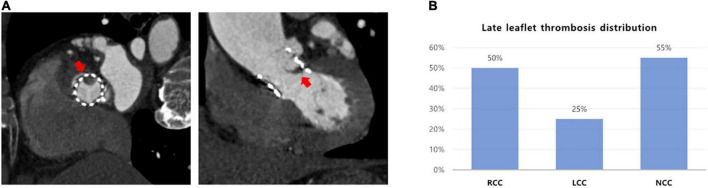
The distribution of subclinical leaflet thrombosis in TAVR. (A) Evaluation of leaflet thickening and reduced leaflet motion based on MDCT. (B) Leaflet thrombosis distribution in cusps. MDCT, multidetector computed tomography; TAVR, transaortic valve replacement.
